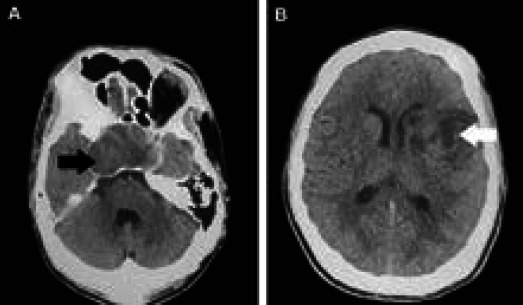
Non-contrast CT scan of the brain.
